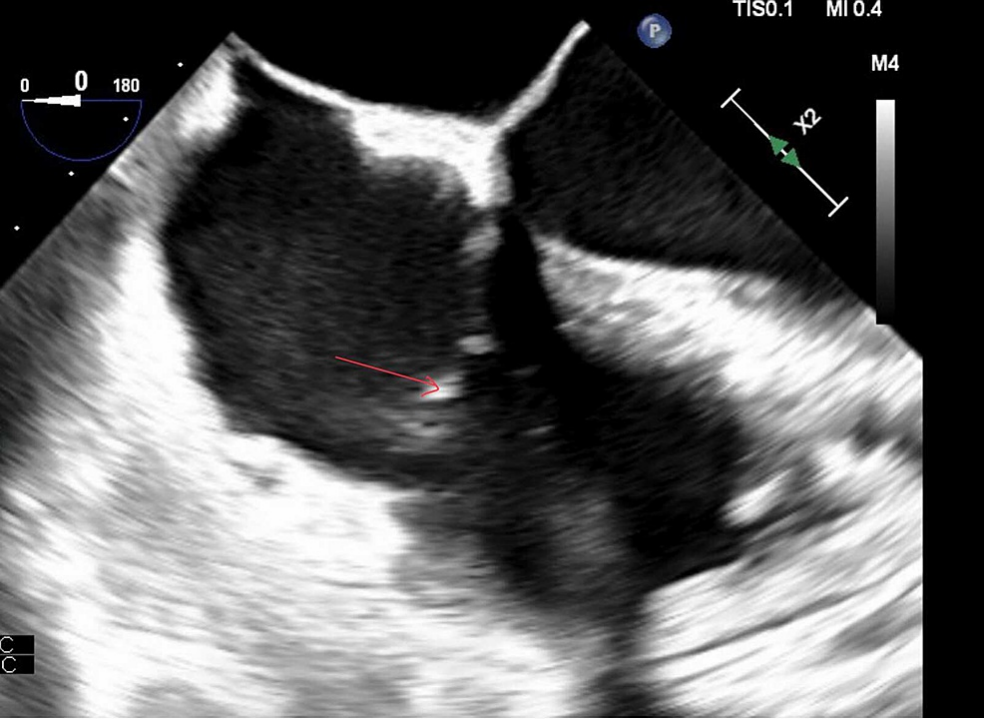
TEE showing significant reduction (>80%) of vegetation TEE: transesophageal echocardiography
